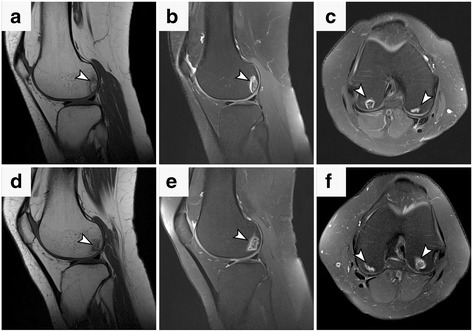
Osteonecrosis of the knees in a 33-year-old woman with SCD. (a - f) T1-weighted (a, d - sagittal) and proton density fat-suppressed (b, e - sagittal; c, f - axial) sequences demonstrates subchondral lesions (arrowheads) on the posterior portions of the right (a, b, c) and left (d, e, f) condyles. The osteonecrotic lesion is surrounded by border appearing hypo/hyperintense (double rim sign) (b, c, e, f) indicating granulation and sclerosis respectively (arrowheads). MRI scans before surgery shows subchondral lesions representing early osteonecrosis in Ficat II stage. Both knees were treated with BMC implantation
